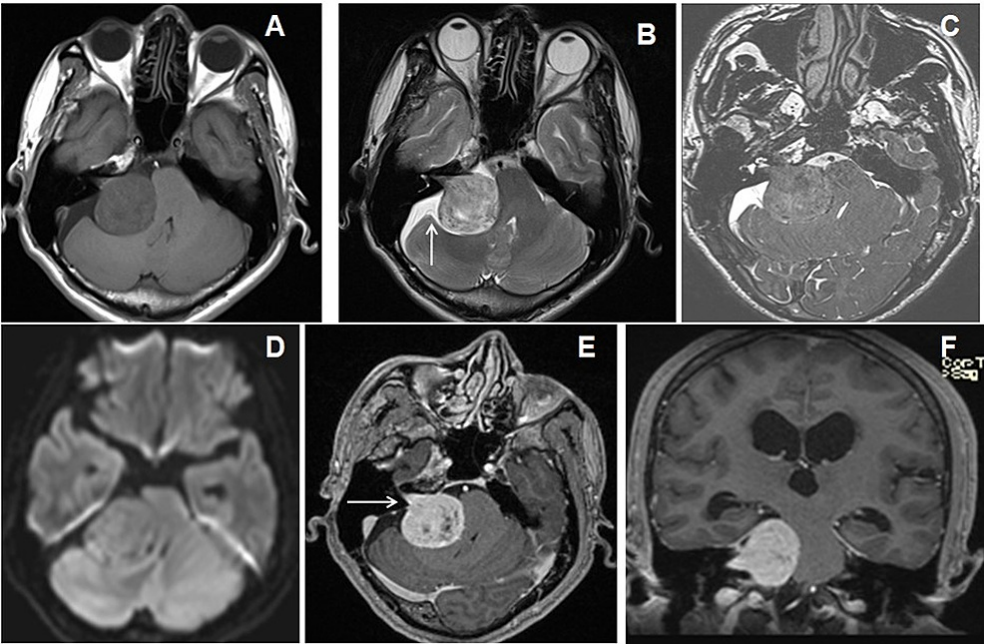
Cranial MRI of a 50-year-old male patient with vertigo and ataxia due to right-sided vestibular schwannoma. (A and B) Axial T1WI and T2WI images show an extra-axial T1W isointense and T2 mixed signal intensity extra-axial lesion in right C-P angle cistern with CSF cleft (↑arrow). (C) 3D-CISS image shows the tumoral extension into the right IAC. (D) DWI image does not show diffusion restriction. (E and F) Axial and coronal T1W post-contrast images show moderate homogenous enhancement of the lesion (→arrow) with fine non-enhancing cystic spaces. CSF: cerebrospinal fluid; 3D-CISS: three-dimensional constructive interference in steady state; DWI: diffusion-weighted image
